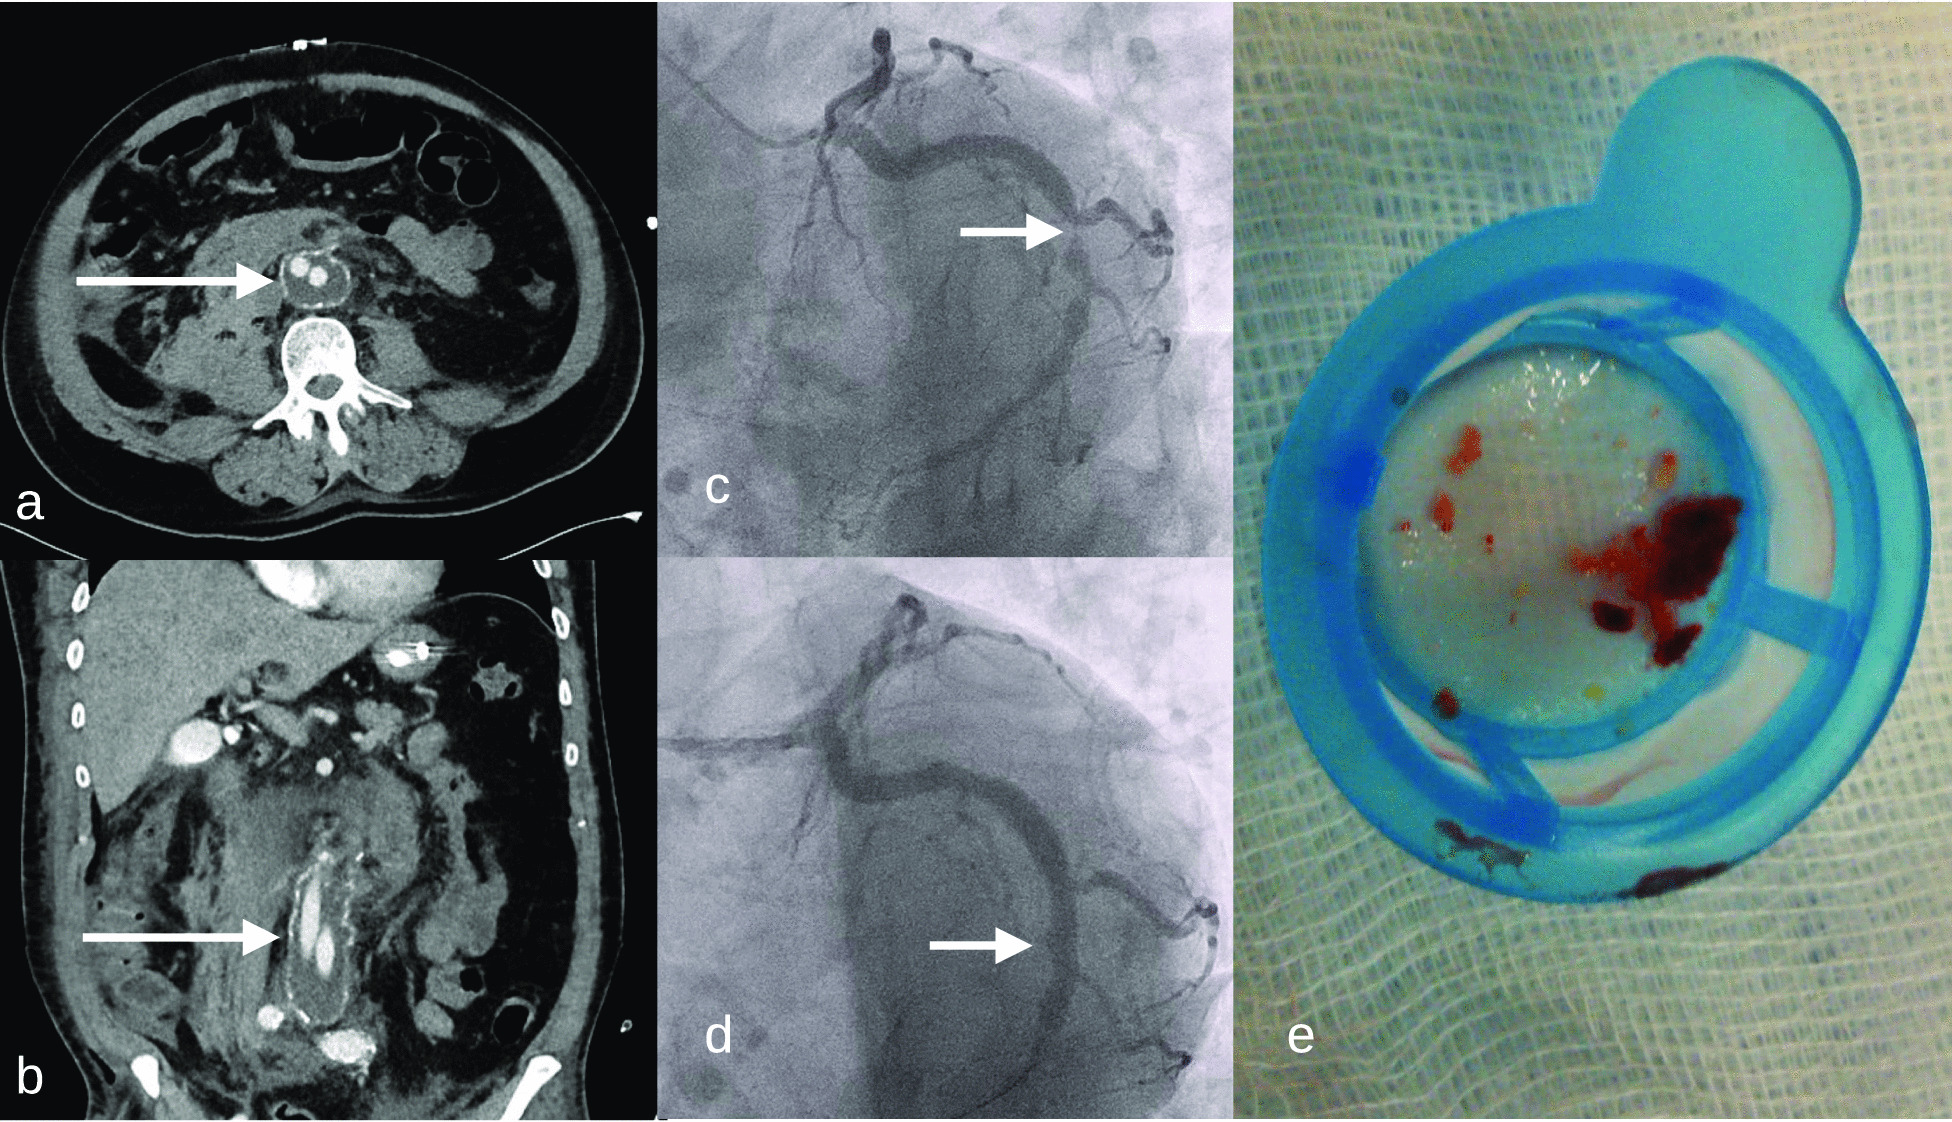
a Aortic aneurysma (long arrow) in CT axial view, b aortic aneurysm (long arrow) in CT coronal view, c coronary angiography showing aneurysmatic RCX (short arrow) before intervention, d coronary angiography with RCX after stent (short arrow) implantation, e the retrieved white thrombi
